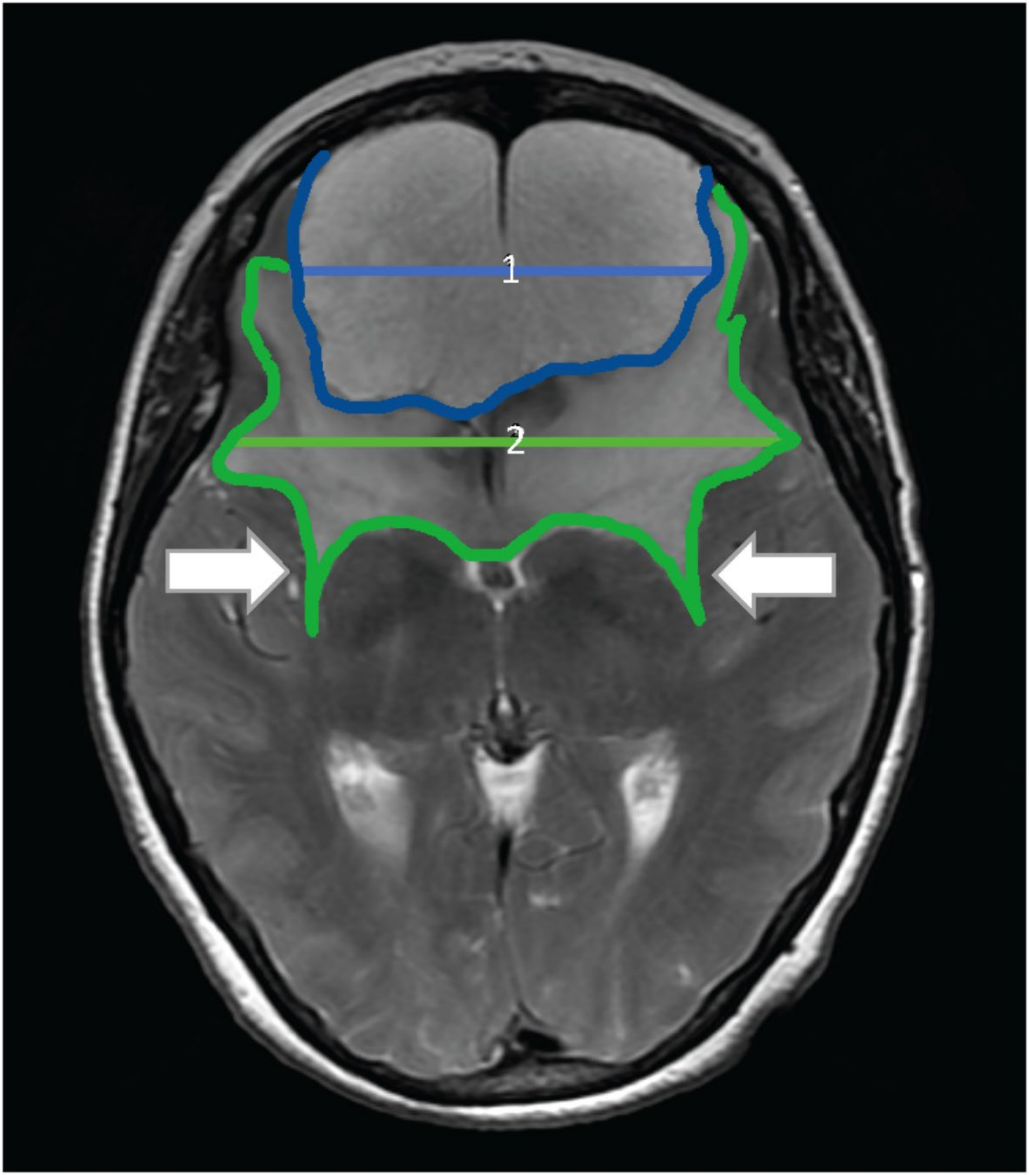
Olfactory groove meningioma with extensive peritumoral edema. T2-weighted MRI sequence of a patient with a large (maximum tumor diameter > 50 mm; 1 = 71 mm; highligthed in blue) olfactory groove meningioma with extensive bihemispheric, peritumoral edema (maximum edema diameter > maximum tumor diameter; 2 = 98 mm; highlighted in green). The white arrow marks the so-called “sabre-tooth” sign^6^, which describes a specific pattern of severe cerebral edema extending posteriorly within the external capsule.
